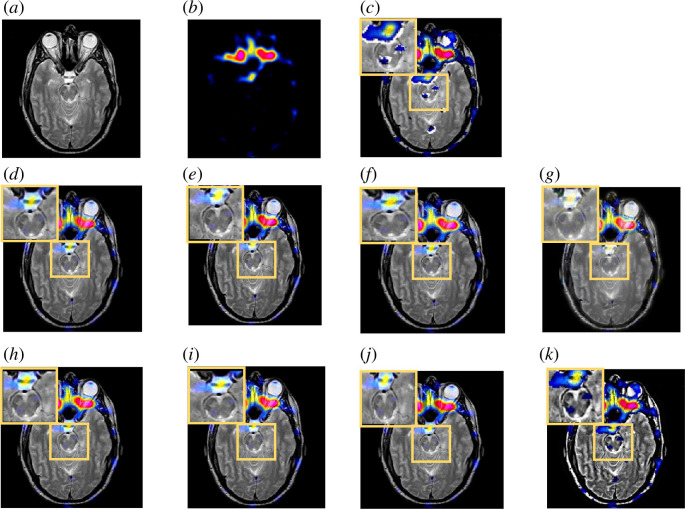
Comparison of fused image on the TITc-SPECT from Glioma image dataset. (*a*) Original MRI image. (*b*) Original SPECT image. (*c*) MLMG-PCNN [16]. (*d*) JBF [18]. (*e*) Local extreme map GF [39]. (*f*) LRD [7]. (*g*) ACOF [40]. (*h*) MDHU [41]. (*i*) FGF [42]. (*j*) Fusion based on phase congruency [43]. (*k*) Proposed.
